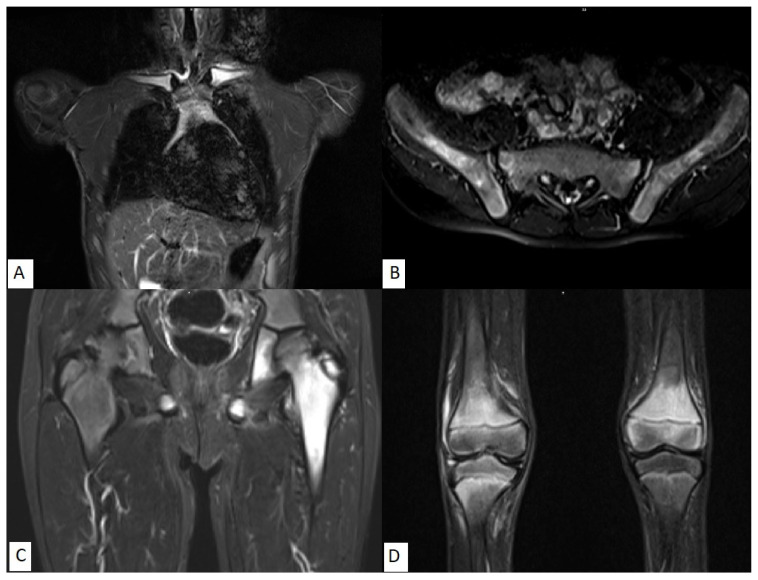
Magnetic resonance imaging of chronic nonbacterial osteomyelitis patients. Hyperintense signal changes in T2-weighted sequences are observed. A) Imaging results of a 17-year-old male patient, in the metaphyseal part of the left clavicle and adjacent soft tissue; B) a 12-year-old female patient, in the right ilium; C) a 13-year-old male patient, in the ischium, greater trochanter, and metaphysis of the left femur; D) a 12-year-old female patient, in the distal epiphysis of the right femur, in the proximal metaphysis of the right tibia, and in the distal epiphysis of the left femur.
